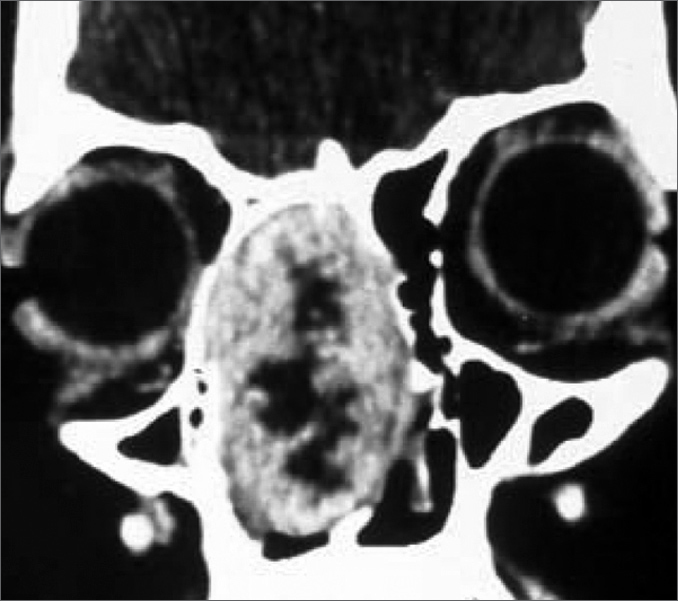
Coronal CT scan image showing a tumor in the right nasal cavity, with intact intracranial and orbital limits.
